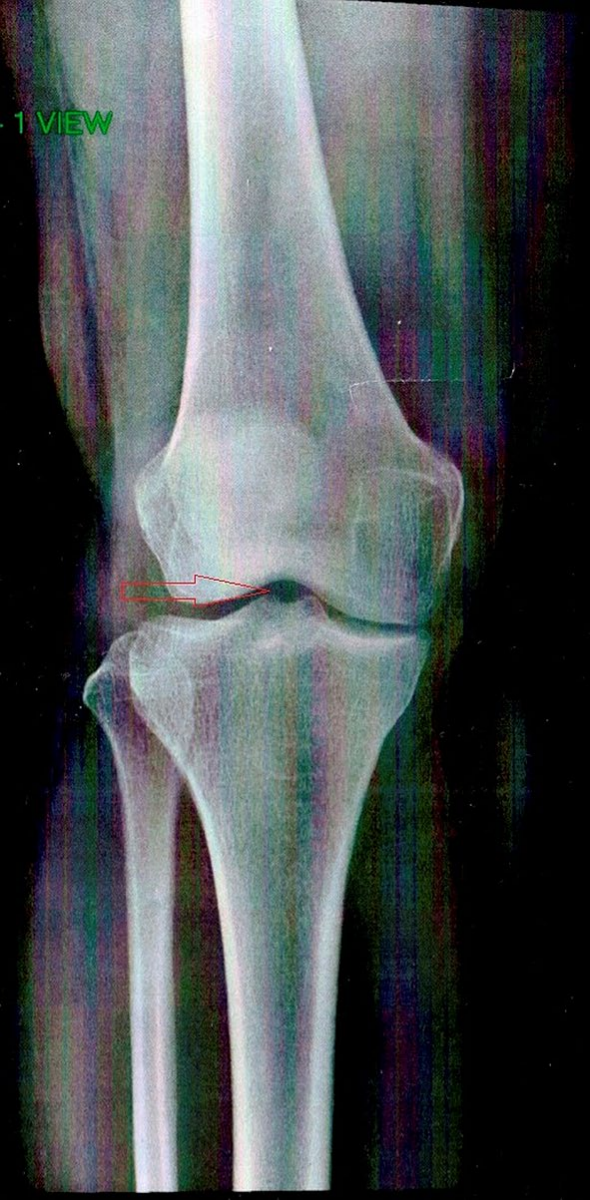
Right knee radiograph: medial compartment tibiofemoral joint space narrowing. Patient presented with dactylitis, intense pain in asymmetrical areas (clavicle, right knee and left ankle) with increased capitation in bone scintigraphy. The right knee radiograph displayed medial compartment tibiofemoral joint narrowing in the right knee, indicated by the *arrow in the figure*, without pathological calcifications or radiographic bone density and texture alterations
